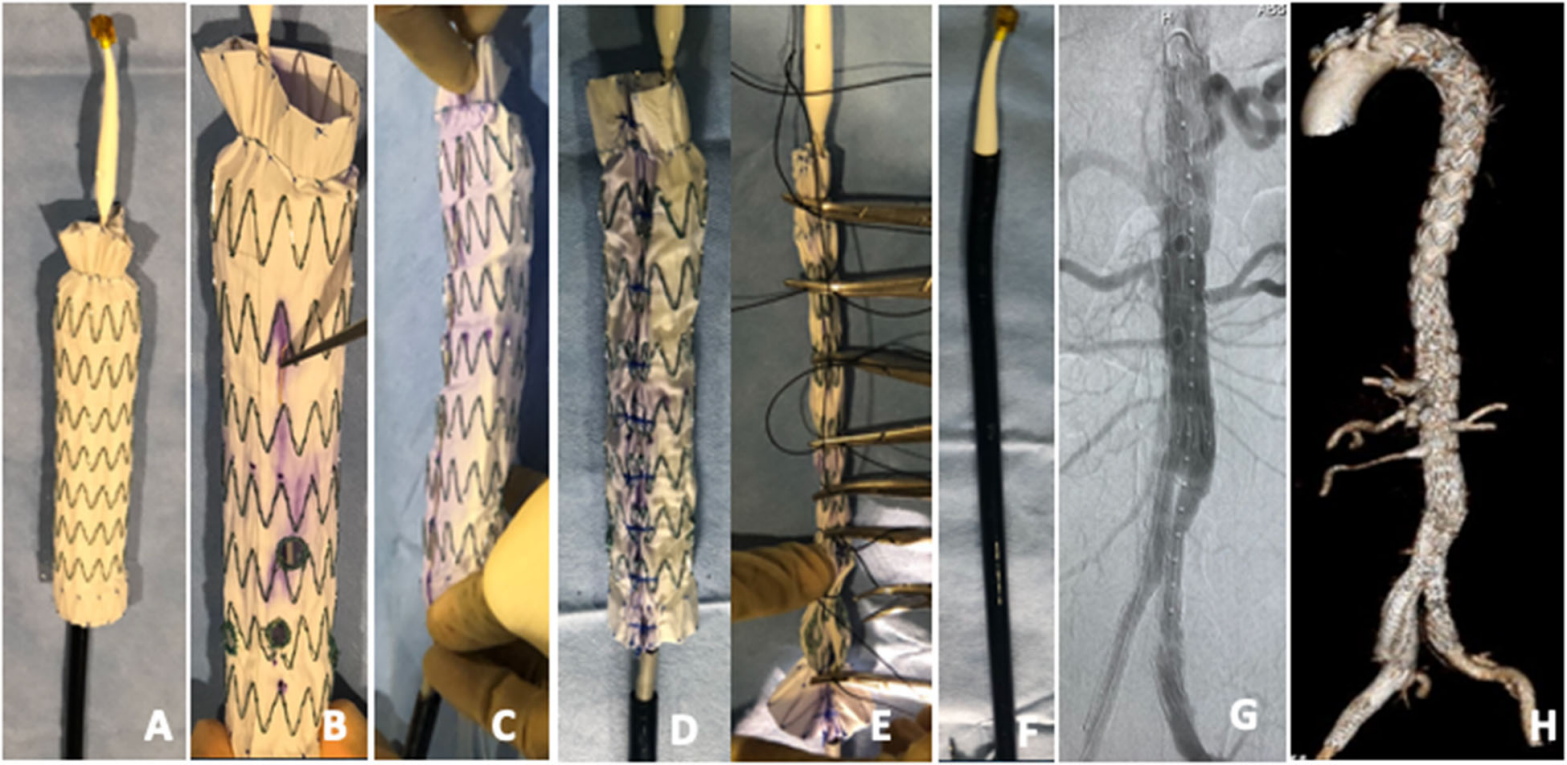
Steps of modification of the Zenith TX2 Dissection Endovascular Graft with Pro-Form. The device is deployed on a sterile back Table (A). Creation and reinforcement of fenestrations are as described in Fig. 5B. Note the presence of an anterior marker (not yet sutured in place) which aids with device orientation (B). A long spinal needle is used to redirect one of the 3 nitinol wires removed from the inner cannula through and through the endograft fabric (C). The device is constrained posteriorly at every Z stent as described in Figure legend 2C (D). The graft is collapsed using silk ties and resheathed (E&F). Completion angiography and post-operative 3D CTA showing exclusion of the aneurysm and patency of all 6 target vessels (celiac, SMA, 2 right renal arteries, 1 left renal artery, and right internal iliac artery) (G&H). Rationale for device selection: The Zenith TX2 double tapered (32–24-158) was chosen for its size and length (32 mm diameter into a 30 mm diameter existing graft and 158 cm long) to allow seal into the existing TEVAR with a minimum of 3 stent overlap while providing adequate room for creation of five fenestrations. The distal tapered (24 mm diameter) allowed for the use of a Zenith fenestrated universal bifurcated device we had available in our inventory
